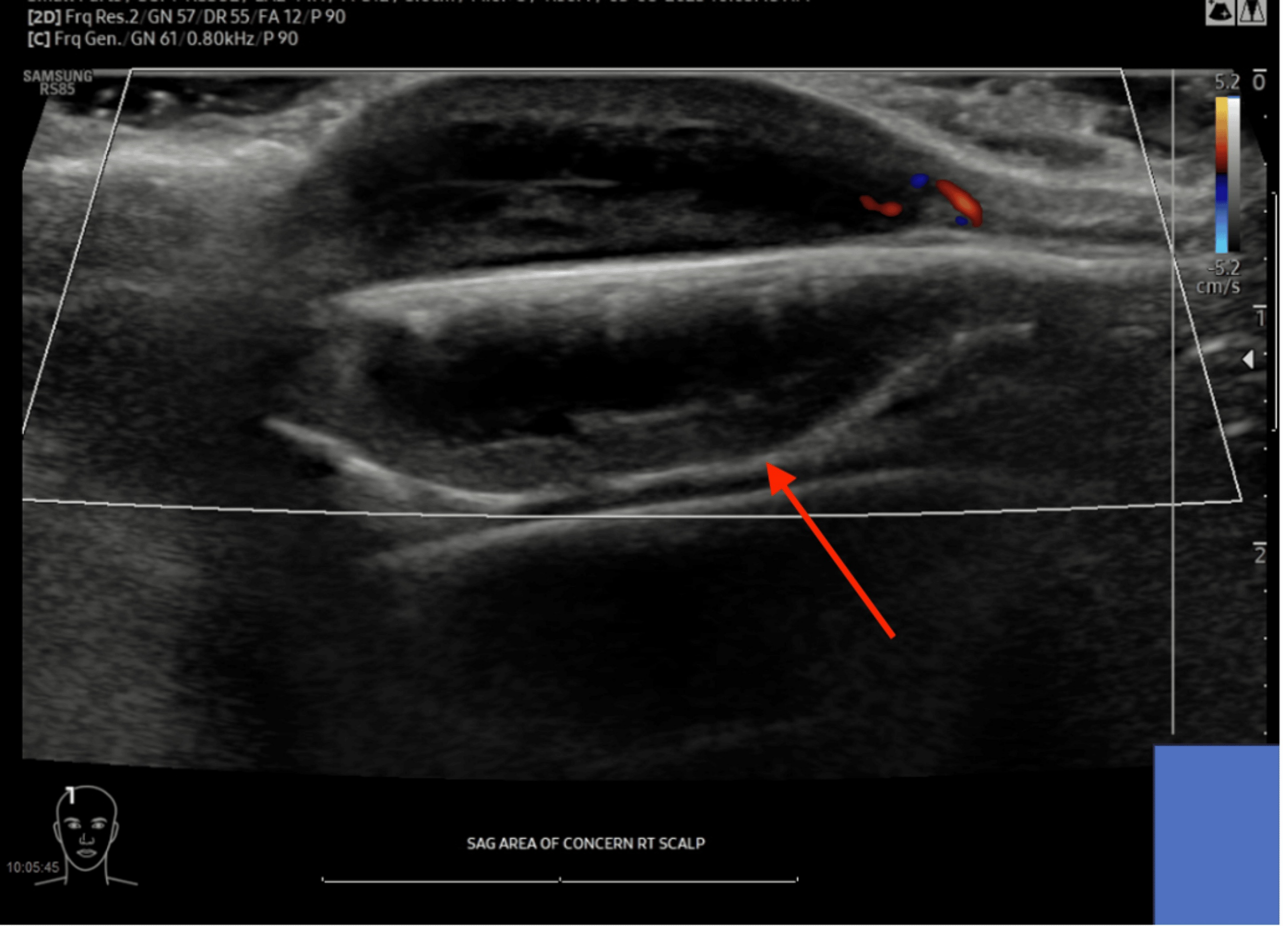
Ultrasound of right frontal mass (arrow), measuring 2.5 cm in length, 2.6 cm in width, and 0.8 cm in depth.
